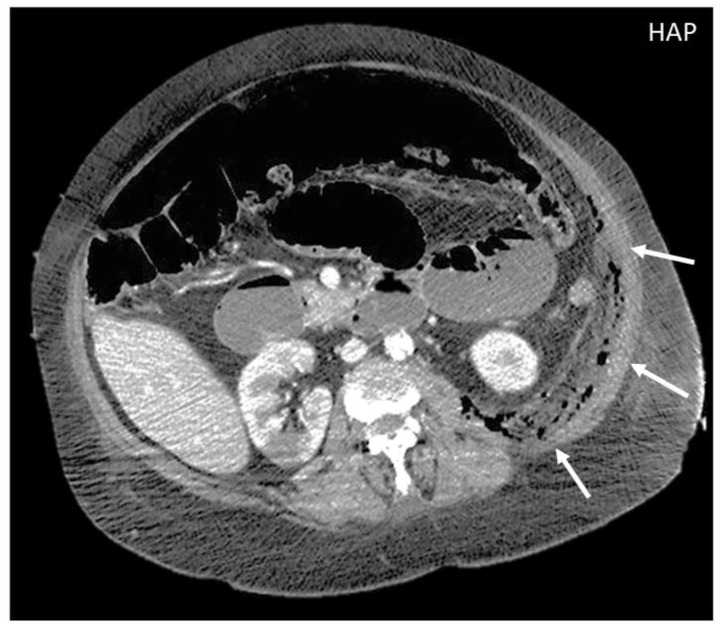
CT appearance of necrotizing fasciitis in a 58-year-old woman with a recent left lower limb open wound who arrived at the ER in septic shock. Arterial phase axial CT scan reveals the presence of free air in the fascial planes of the left lateral and posterior abdominal wall, with fascial thickening and lack of muscular enhancement, as compared to its counterpart (arrows). These elements are suggestive of necrotizing fasciitis. The patient was promptly referred to surgery, but she died on the operatory table.
